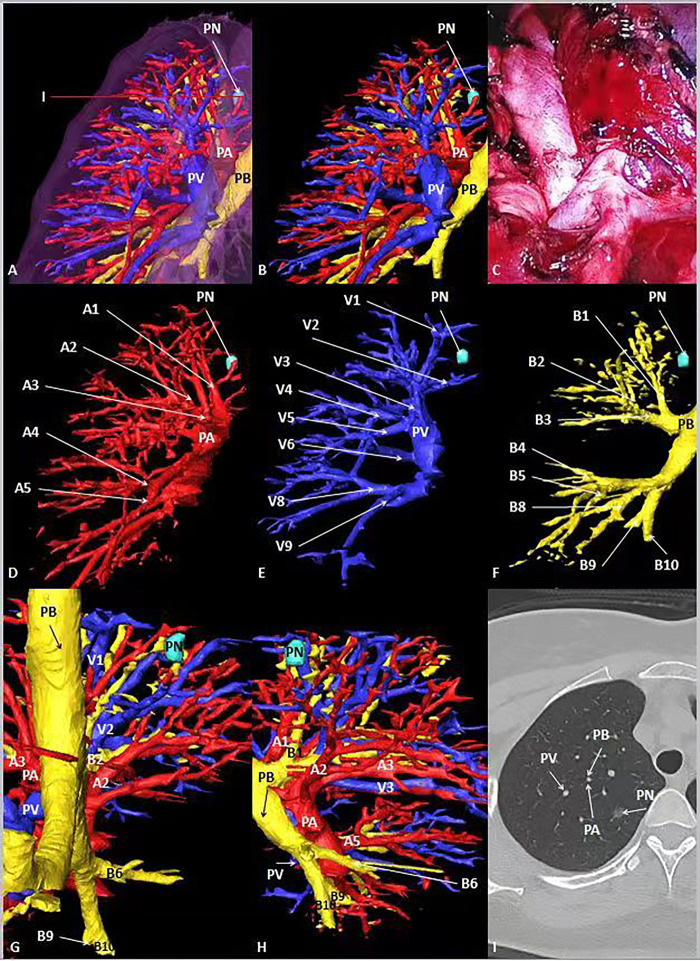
General CT, 3D-CT of the patient. (A, B) 3D-CT of the patient shows the location of pulmonary nodules in the right upper lung. (C) Intraoperative condition. (D–F) 3D-CT shows the position between target pulmonary nodules and surrounding pulmonary arteries, veins and bronchi. (G, H) Observe the position between target pulmonary nodules and surrounding pulmonary arteries, veins and bronchi from different perspectives. (I) The position between the target pulmonary nodule and the surrounding pulmonary artery, vein and bronchus on the General CT.
